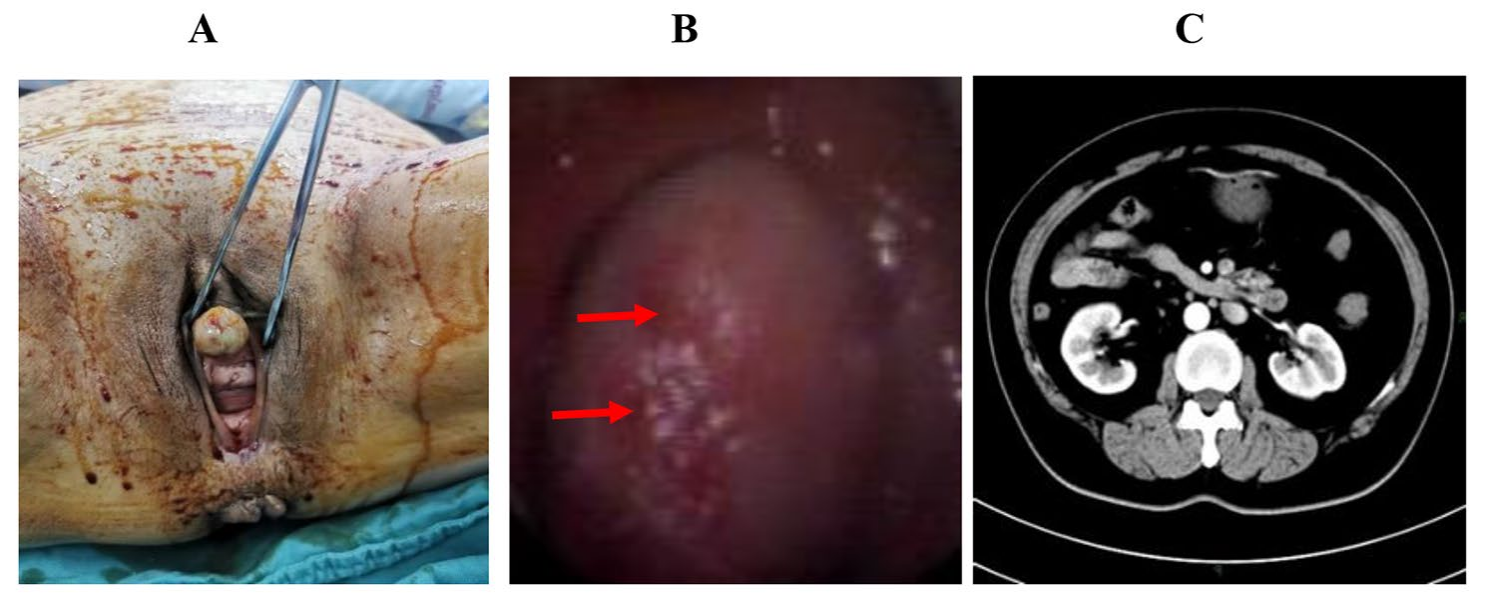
Pre-operative examination. A The gross appearance of an external urethral synovial sarcoma; measuring approximately 3.0 cm × 4.0 cm, with completed coat on the external genitalia. B Cystoscopy revealed: a large mass measuring approximately 2.5cmx2.0 cm with ulceration and oozing on the right side of the external urethral opening. C Plain CT scan shows no bilateral ureteral dilatation and no dense shadows in the bilateral ureteral travel area
